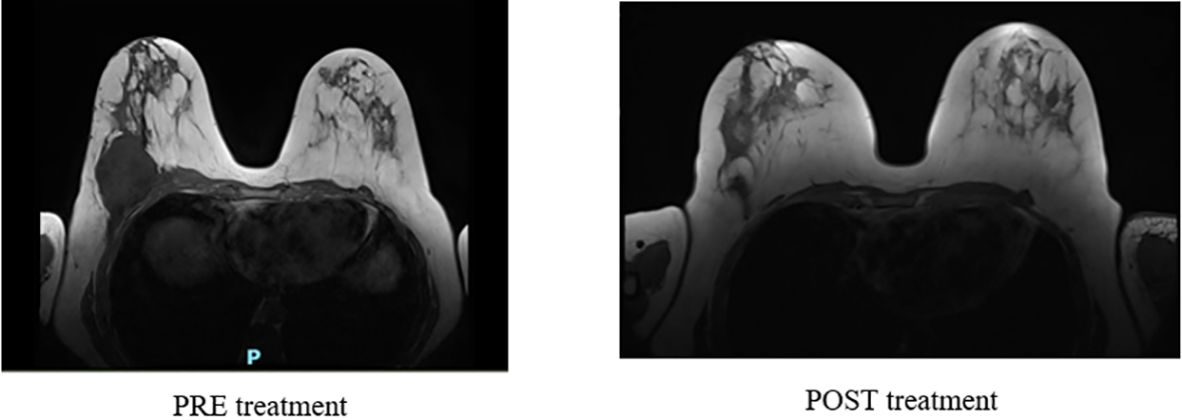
Breast MRI performed before and after neoadjuvant treatment.
